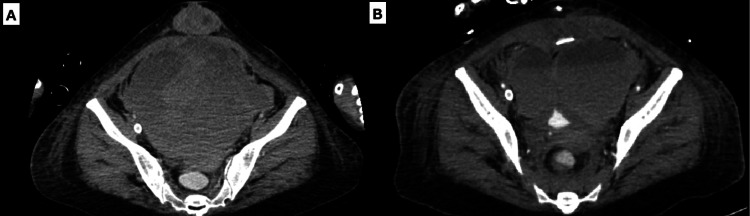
Postoperative hematoma on ECMO with abdominal CT A: On POD 9, a hematoma had formed. The size of the hematoma was approximately 15 cm. We were not able to identify the culprit blood vessels using abdominal contrast-enhanced CT. B: On POD 10, a hematoma formed again. We were able to identify the culprit blood vessels using abdominal contrast-enhanced CT. ECMO, extracorporeal membrane oxygenation; CT, computed tomography; POD, postoperative day
